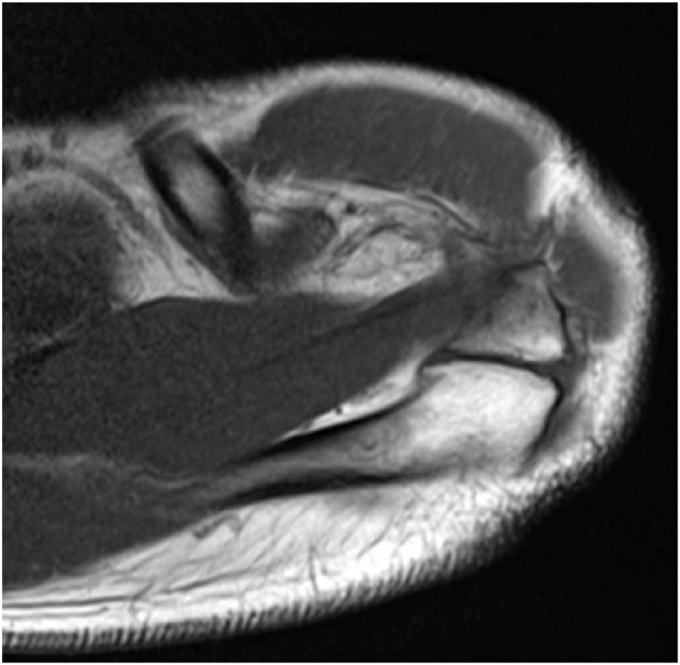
Os acromiale as seen on a T1-weighted magnetic resonance imaging axial view image.
